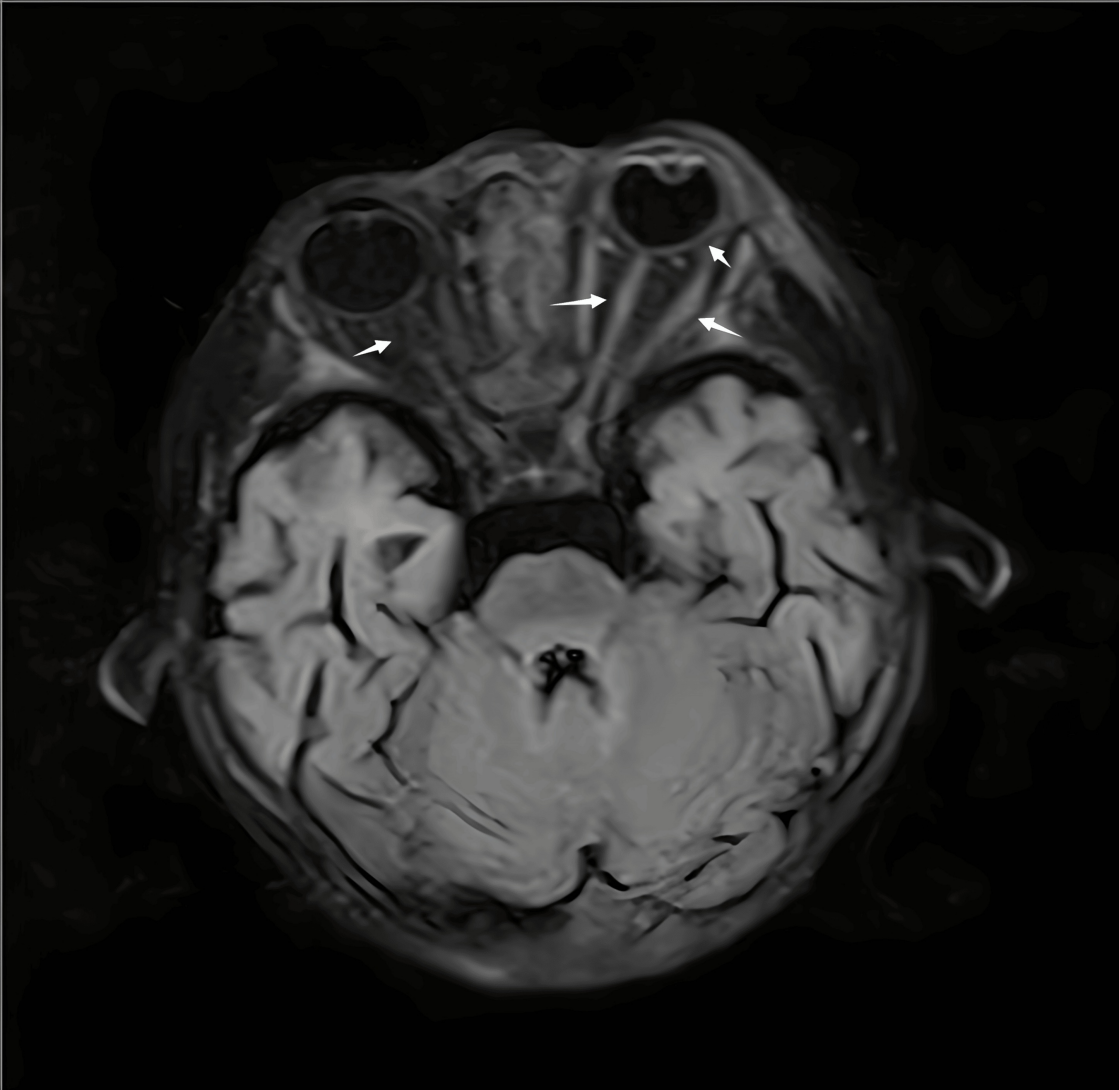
Orbital nuclear MRI on September 6, 2022 This figure presents an orbital MRI utilizing T2-weighted FLAIR imaging. The horizontal scans revealed thickening and increased signal intensity in the eye ring, extraocular muscles, and optic nerve (white arrows). MRI: magnetic resonance imaging, FLAIR: fluid-attenuated inversion recovery
